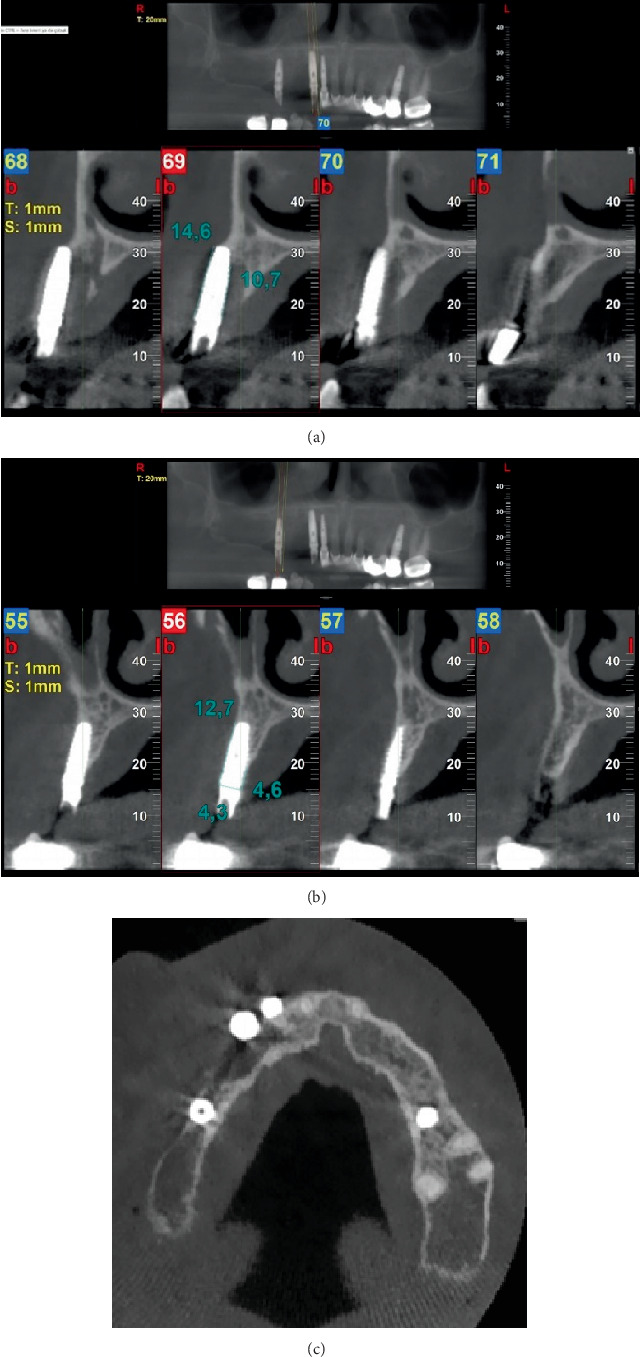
(a) CBCT images of implant #13. (b) CBCT images of implant #15. (c) Axial CBCT image of the related region.
